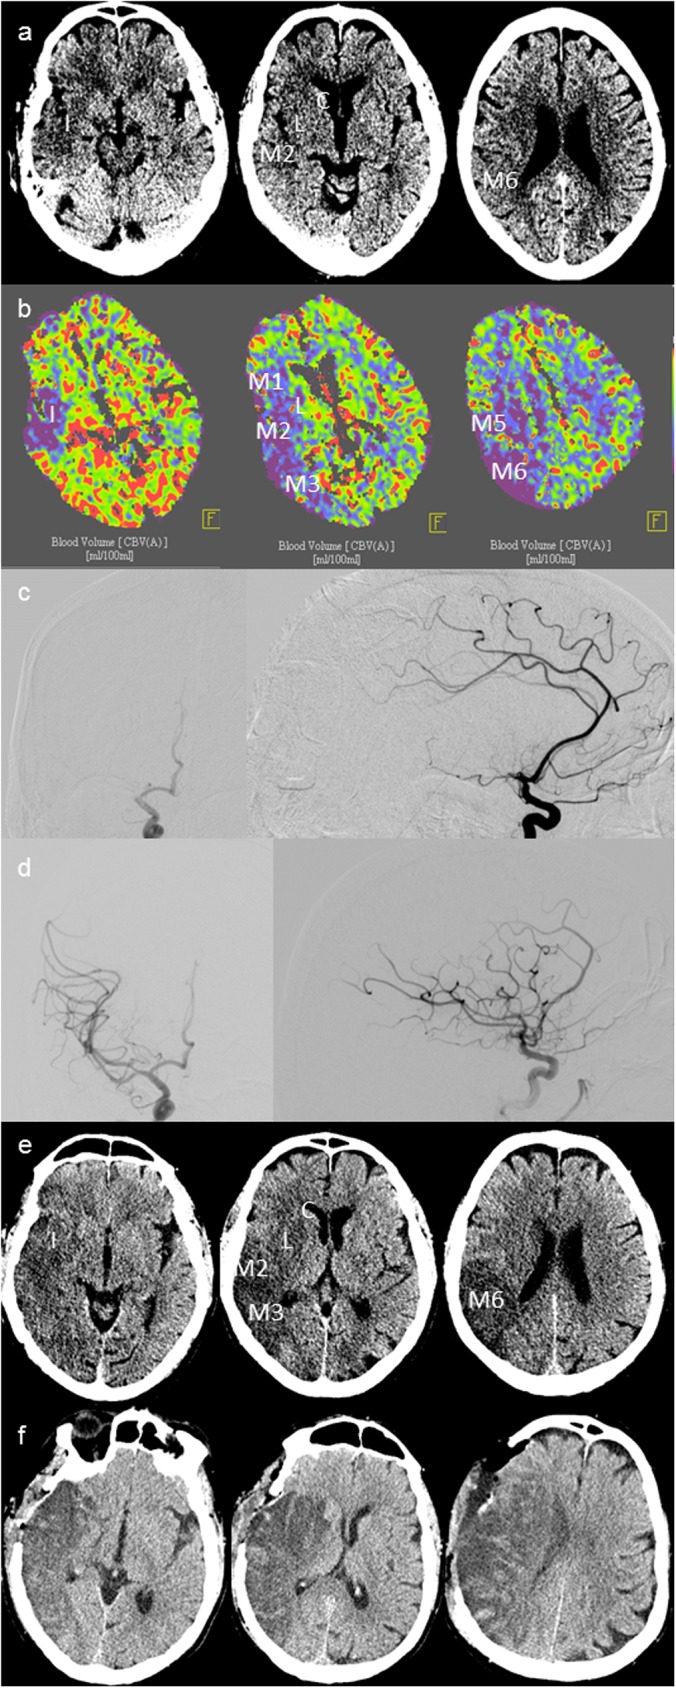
Multimodal stroke imaging from a patient with endovascular therapy and decompressive hemicraniectomy CT-slices are shown in insular (left), ganglionic (middle) and supraganglionic levels from left to right. (a) ncCT on baseline presentation with an ASPECTS of 5, a symptom-to-CT time of 118 min and a baseline NIHSS of 12. (b) Cerebral blood volume on baseline with an ASPECTS of 3. (c) DSA before EVT with mTICI 0 and (d) after EVT with successful recanalization. (e) follow up ncCT with temporal edematous swelling compressing the right anterior horn of the lateral ventricle. (f) ncCT after DH with infarct demarcation of the territorial infarction of the medial cerebral artery circulation. ncCT: non-contrast cranial computed tomography; ASPECTS: Alberta Stroke Program Early CT score; NIHSS: National Institute of Health Stroke Scale; DSA: digital subtraction angiography; EVT: endovascular therapy; mTICI: modified thrombolysis in cerebral infarction score.
